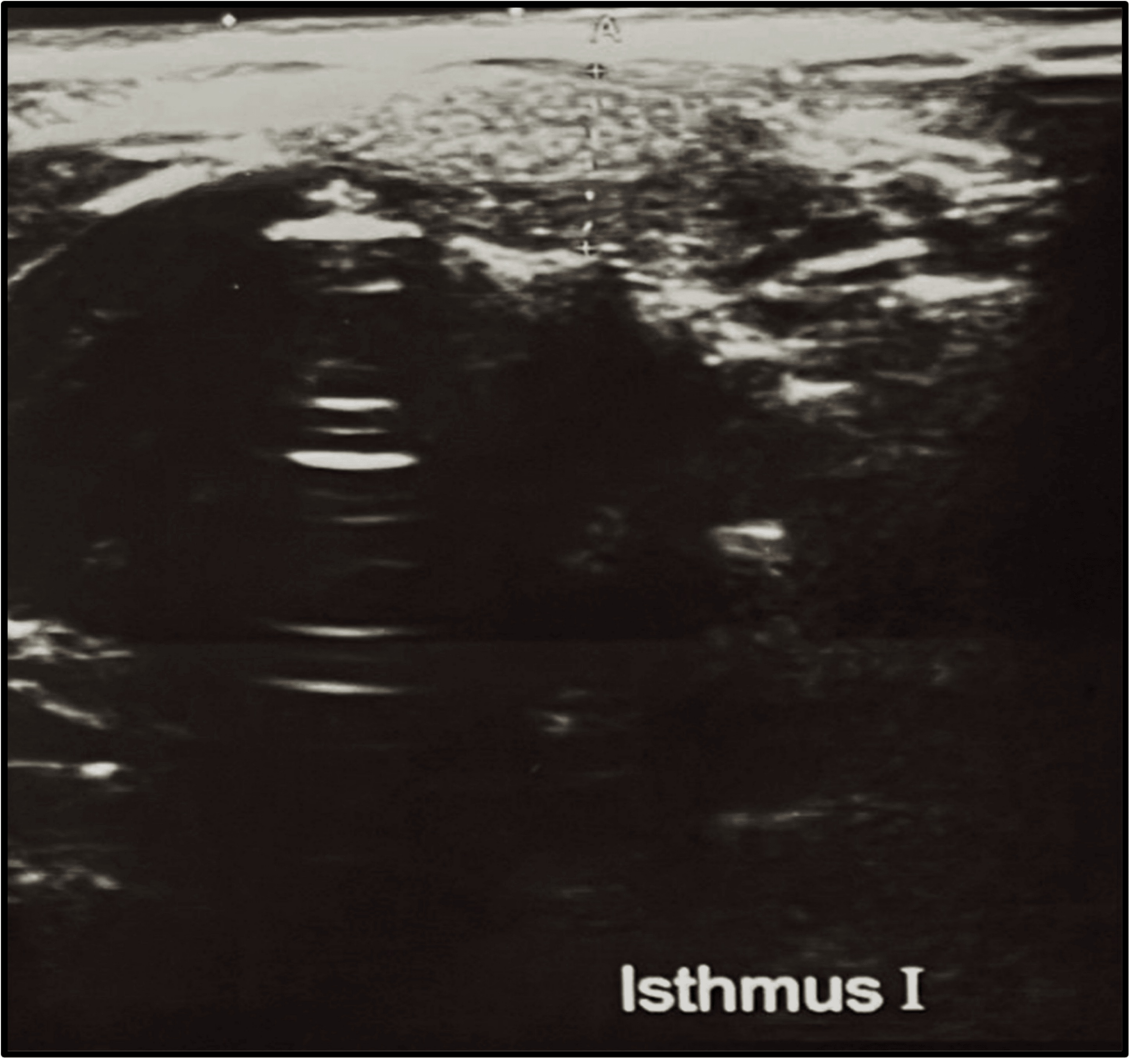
USG thyroid showing thyroid remnant at the level of isthmus (post-total thyroidectomy) USG: ultrasonography
